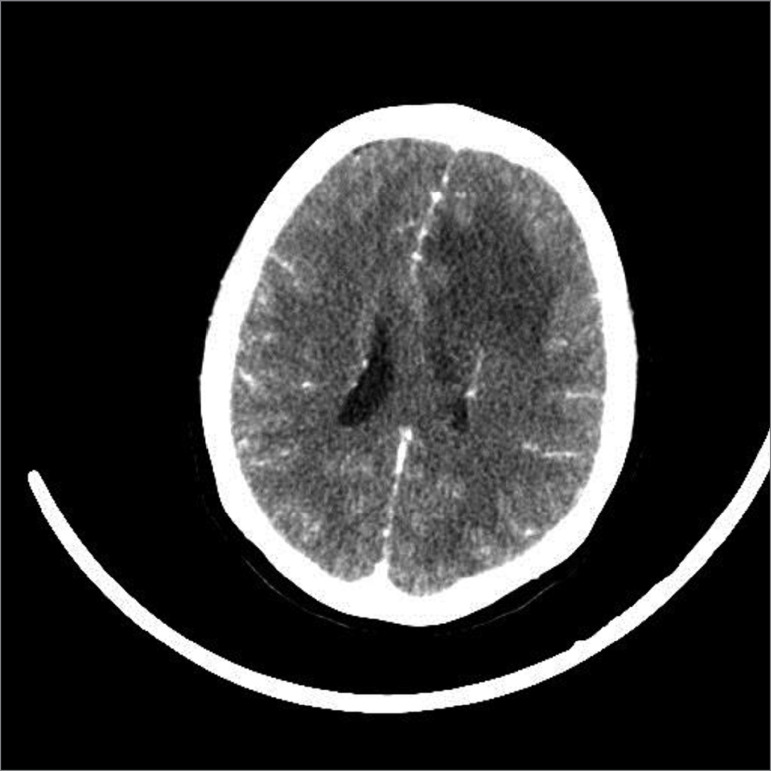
Contrast-enhanced CT scan of the skull. Hypoattenuation area in the left frontal white matter, accentuating the expansive effect on adjacent structures, measuring about 7.0 x 5.0 cm.
